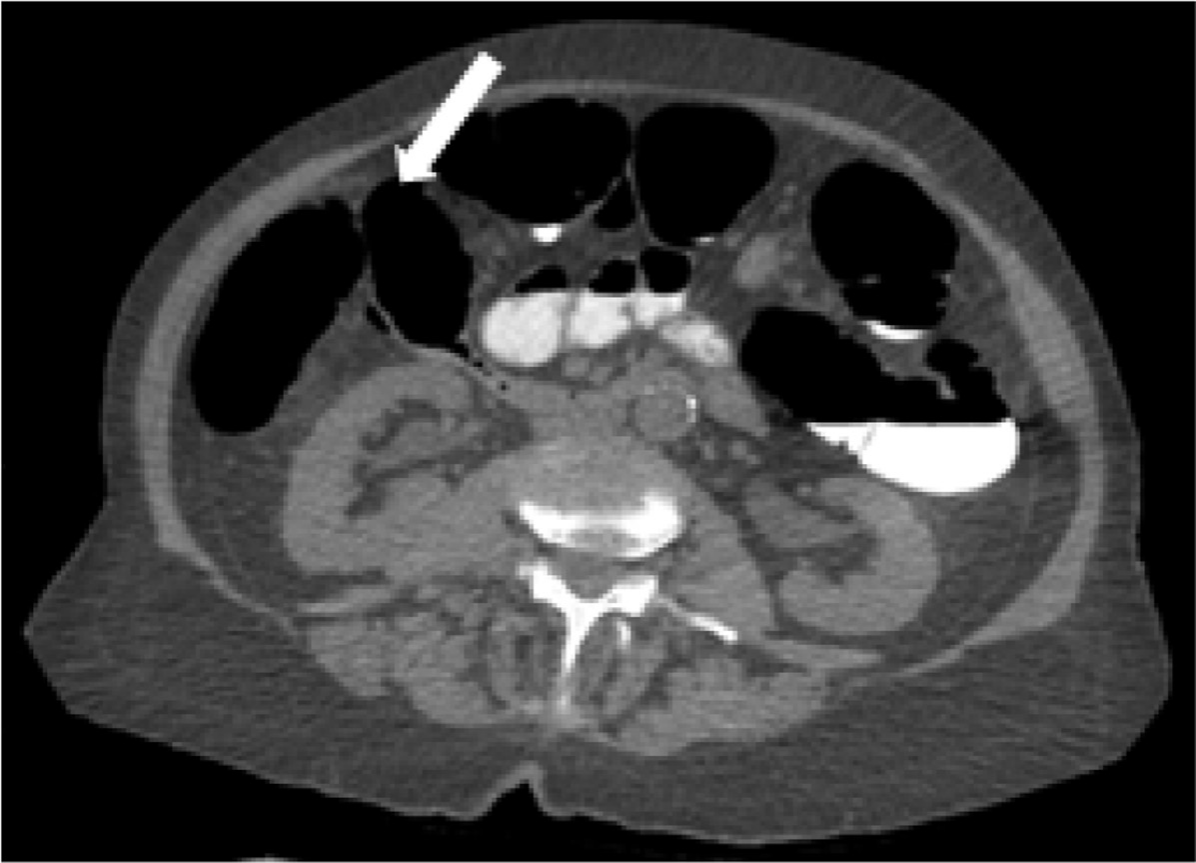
CT virtual colonoscopy study 3 months prior to this admission shows the giant duodenal diverticulum (arrowed) without any surrounding inflammatory stranding.
